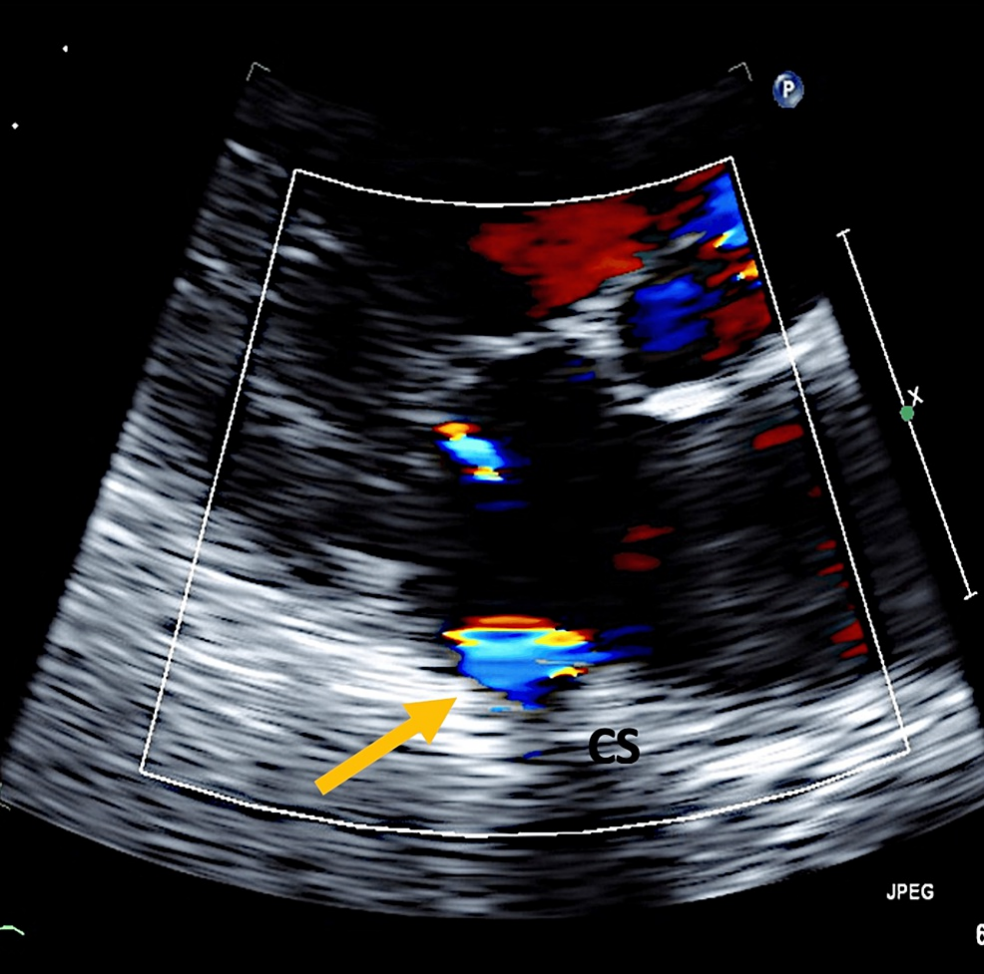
Echocardiogram showing coronary sinus fistula to the right ventricle (yellow arrow). CS: coronary sinus.
